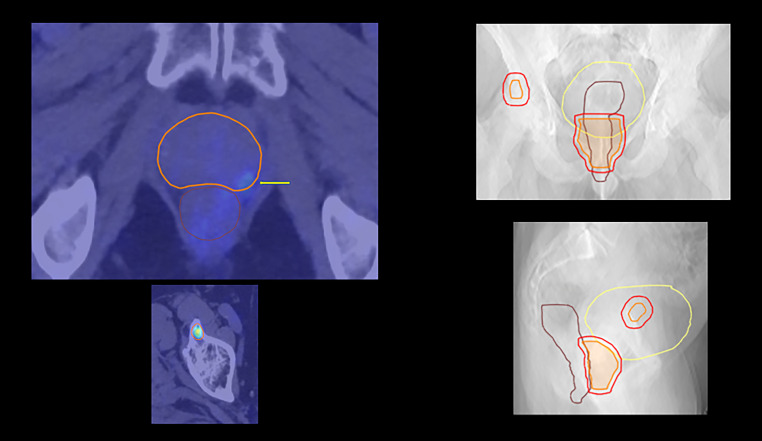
Type 1 reirradiation is a new course of radiotherapy that has geometrical overlap with the irradiated volume of previous courses (left upper panel: prostate reirradiation to a biopsy-confirmed ^68^Ga-PSMA positron emission tomography (PET) positive (*yellow arrow*) relapse after previous external beam radiotherapy), and type 2 is a new course with concerns of toxicity from the cumulative doses but in which there is no overlap with the irradiated volume of previous courses (other panels, treatment planning computed tomography with fused PET in the same patient). Irradiating the single bone metastasis might increase the risk of bladder or bowel toxicity. In the absence of toxicity concerns, the term “repeat organ irradiation” is recommended. *CTV* clinical target volume: *orange*, *PTV* planning target volume: *red*, rectum: *brown*, bladder*: yellow*
